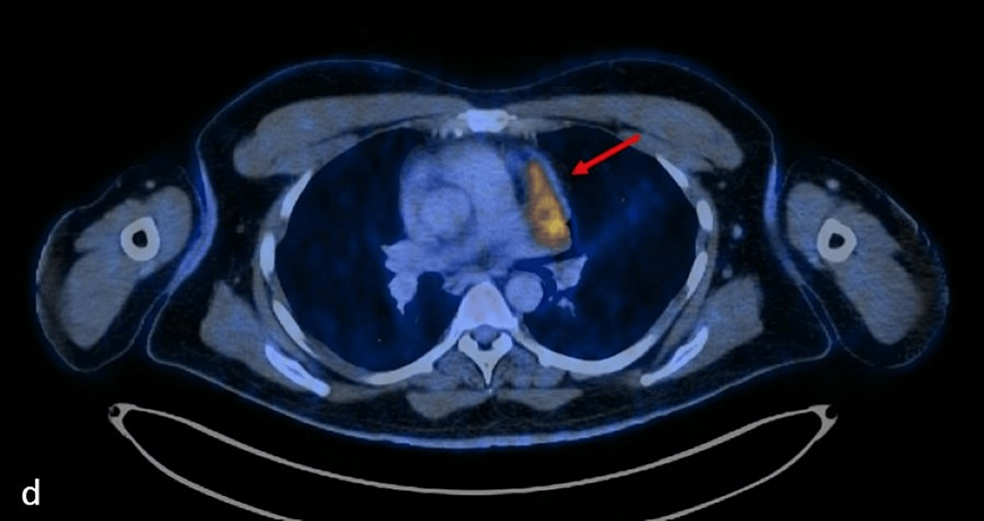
Axial-fused positron emission tomography–computed tomography (PET CT) image of the chest at the level of the pulmonary artery (c,d) show the lesion abutting the main pulmonary artery and left pulmonary artery with loss of fat planes
